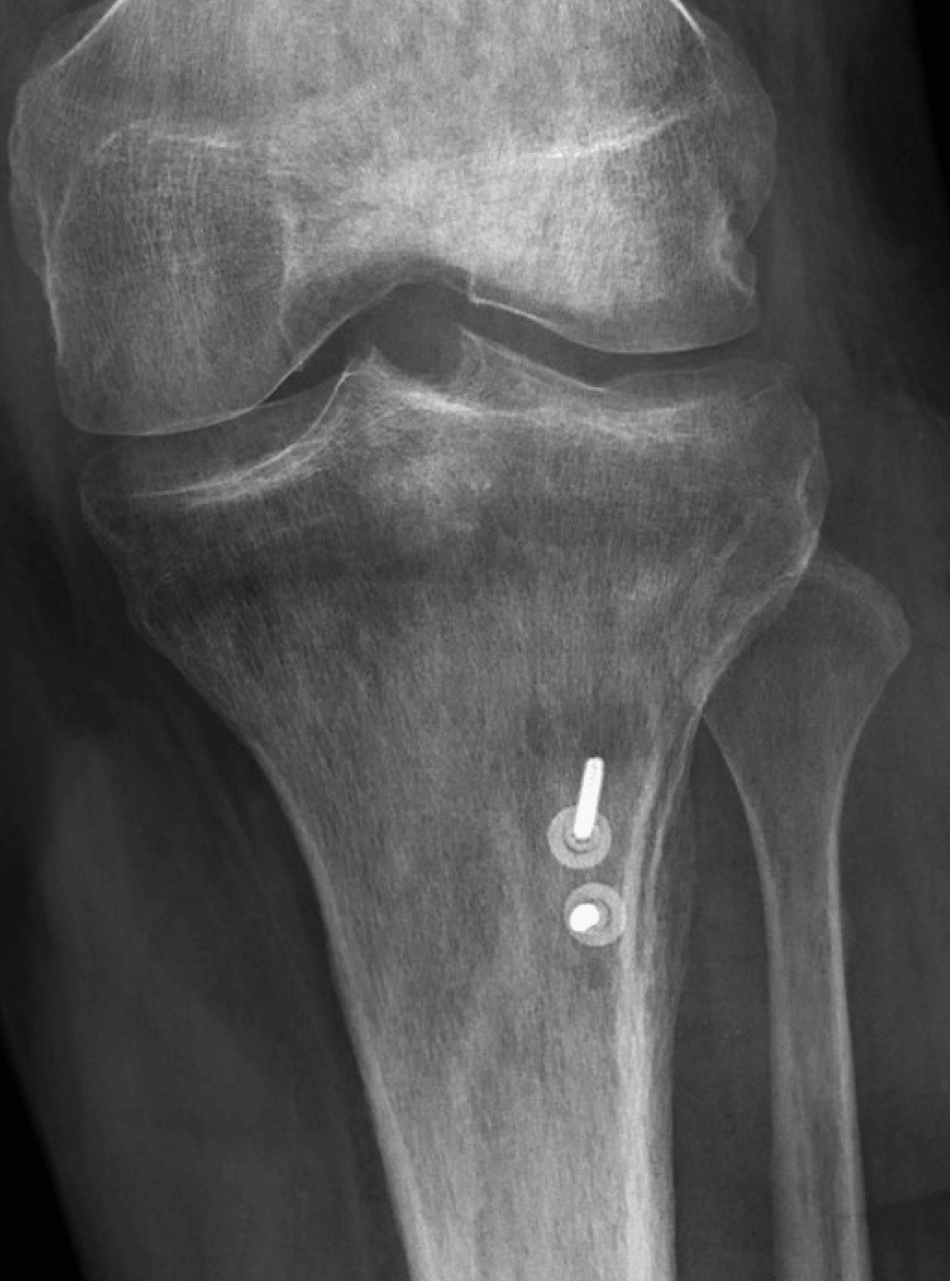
Eight-month AP radiograph of the left tibia demonstrating bony incorporation of calcaneus bone block allograft AP: anteroposterior
